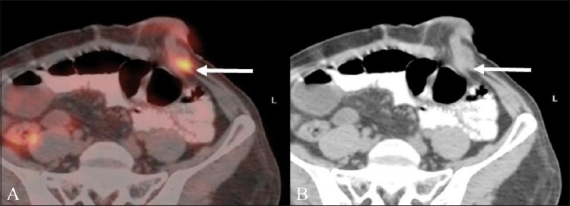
A 59-year-old man who underwent an abdominoperineal resection for low rectal cancer came for follow-up. An FDG PET / CT image (A) reveals a focus of abnormal FDG uptake at the colostomy site (arrow). Close inspection of the CT scan (B) reveals an inconspicuous soft tissue nodule (arrow) corresponding to the increased FDG uptake, suggesting recurrence at the colostomy site. Postoperative changes in the anterior abdominal wall can often mask such recurrent nodules, which are detected on PET, by virtue of their hypermetabolism.
